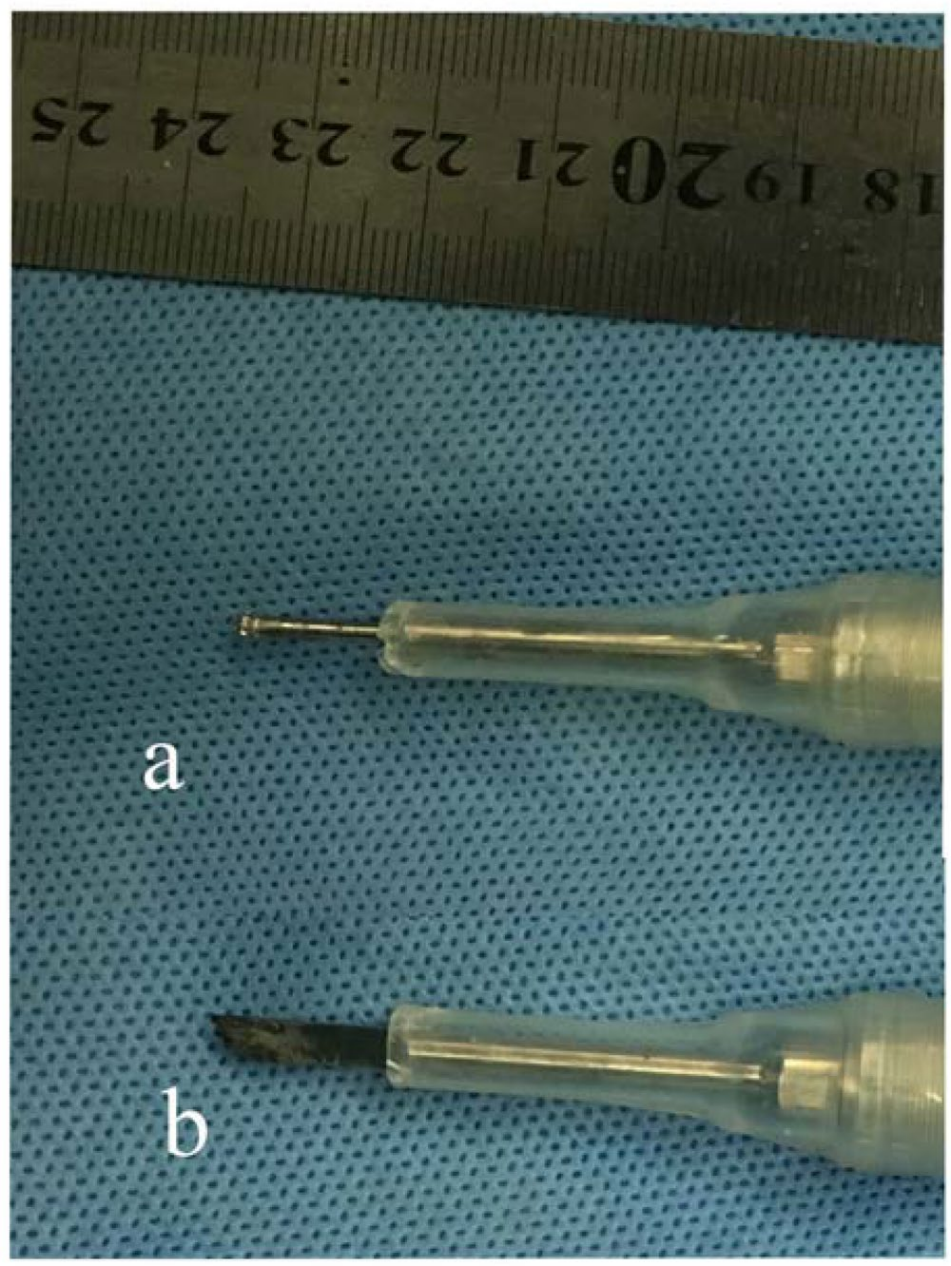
Ultrasonic device tips used for laminectomy. Figure 1a demonstrates a newly designed burr tip used to widen the cutting corridor and decrease the thickness of lamnae to be addressed, it’s especially feasible for cases with ossified ligamentum flavum; b shows a thin scalpel tip designed for osteotomy.
